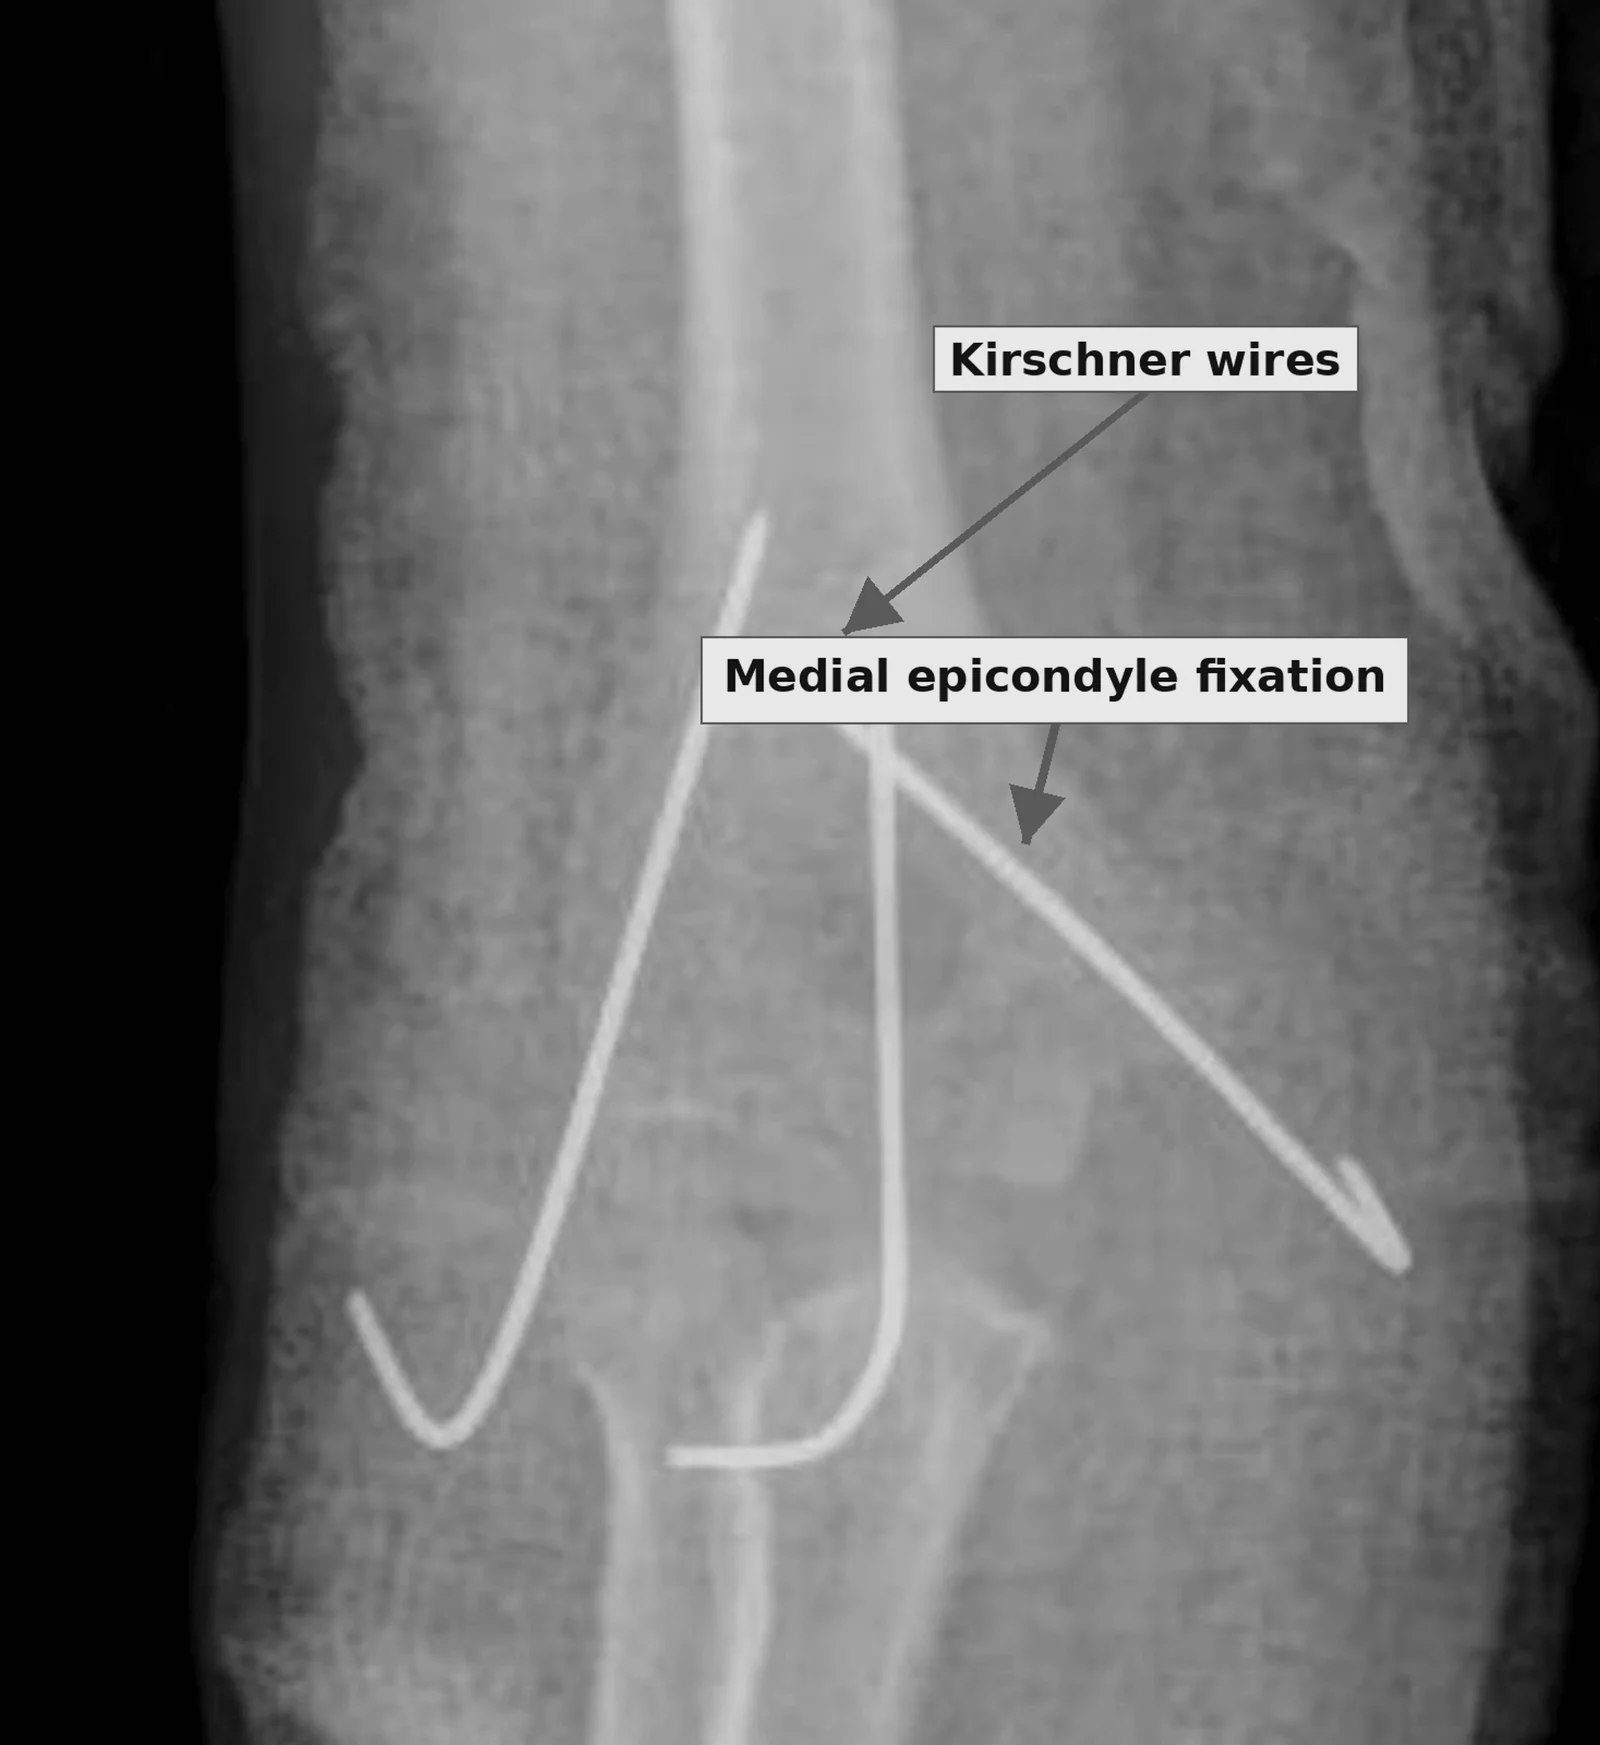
Anteroposterior radiograph of the right elbow following open reduction and internal fixation confirming Kirschner wire placement and anatomical fixation of the medial epicondyle and humeral condyle fragments.
